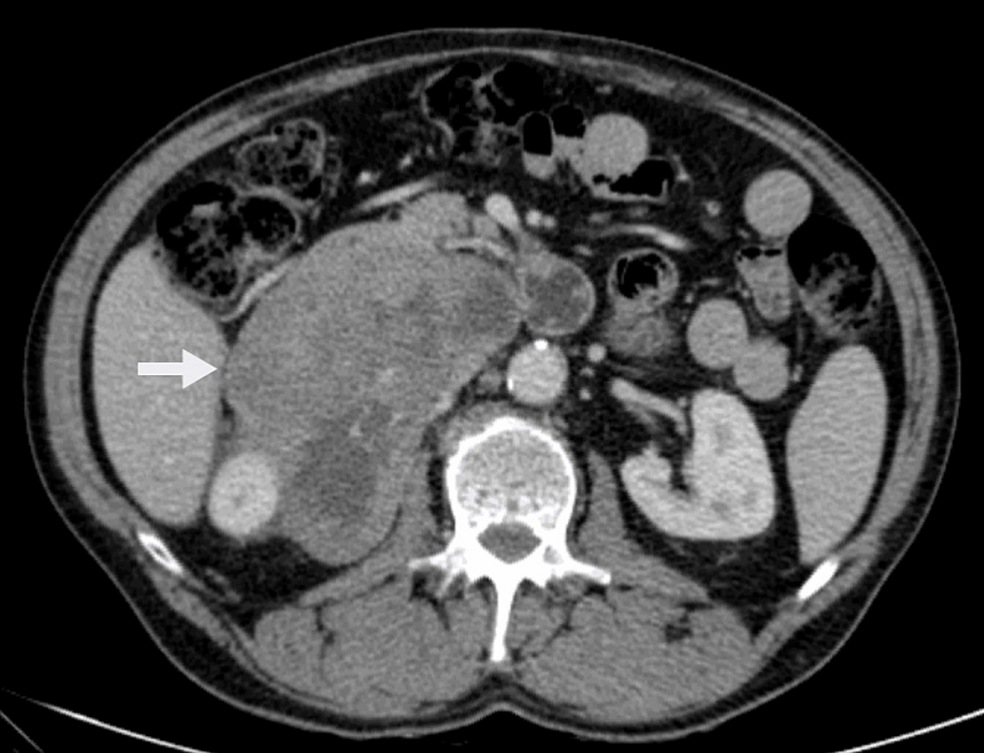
Local recurrence of GIST on the second duodenal portion on CT scan with an apparent invasion of the right kidney GIST, gastrointestinal stromal tumor; CT, computed tomography
